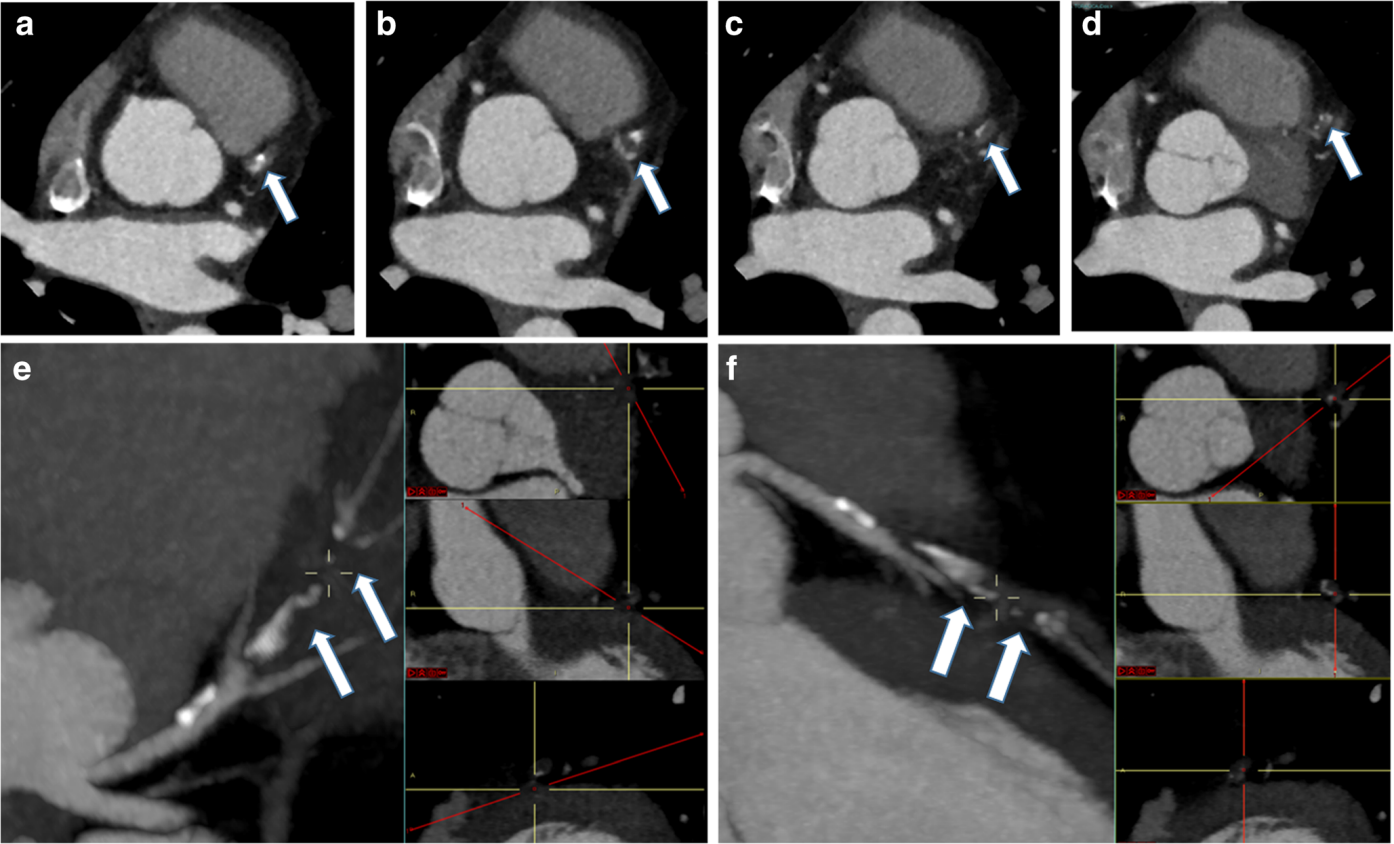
Axial (a–d) and MPR-MIP (e, f) CT images from a patient with obstructive CAD on left anterior coronary artery (arrows). As in Fig. 2, on the right portion of e–f images, the orientation along the three major anatomic planes (axial, coronal, and sagittal) is also showed (red lines)
